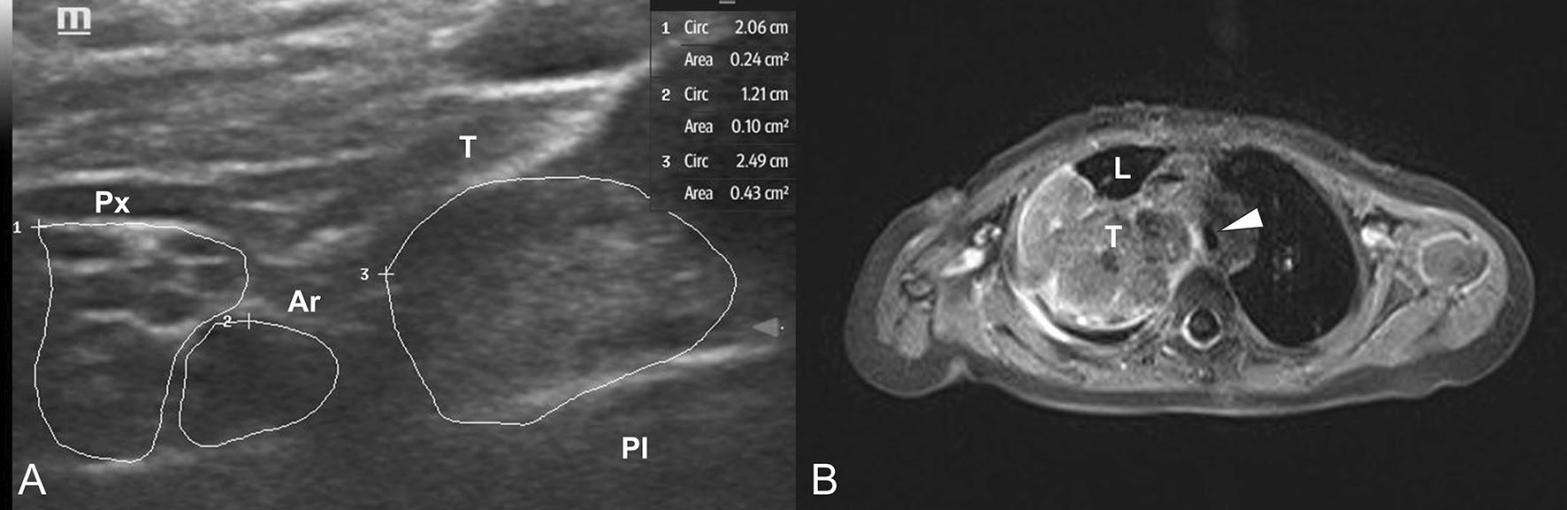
(A) Short-axis from the supraclavicular fossa including all cords of the brachial plexus (Px) with its honey comb aspect, next to the subclavian artery (Ar). The tumor mass (T) (hyperechoic, no fascicular structure in it) is well distinguishable by ultrasound, however owing the high resolution the real distance of the tumor and nerve is below 5 mm; (B) Axial view of the MRI imaging of the same patient showing the intrathoracic extension of the tumor (T) with infiltration of the trachea (white arrow)
